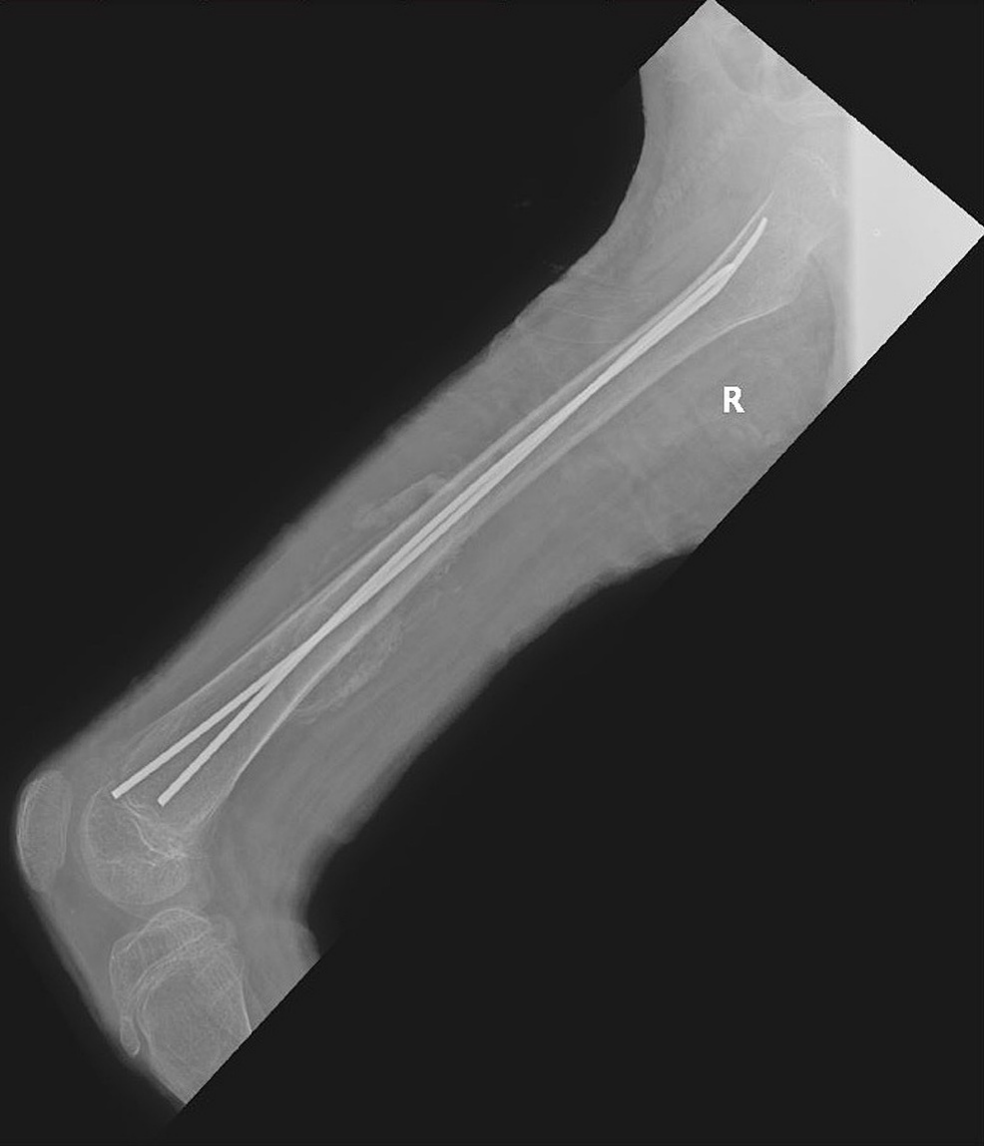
Lateral X-ray image showing acceptable sagittal reduction with good bone healing.
